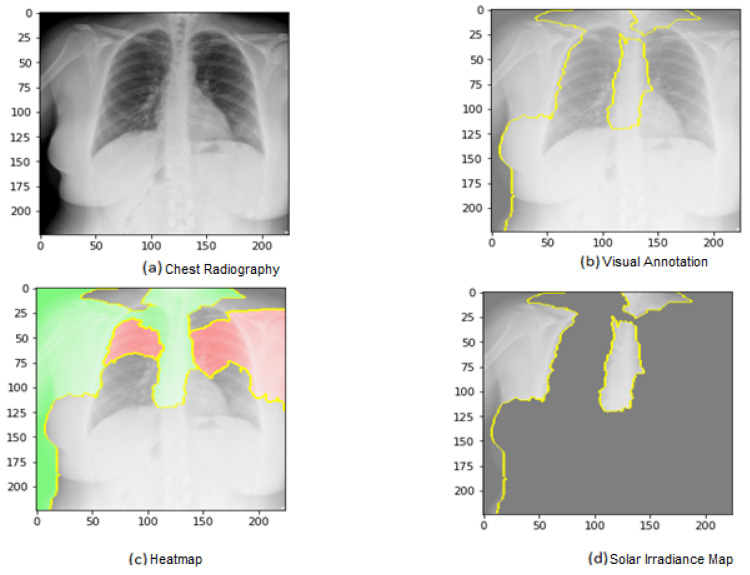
Explainability of X-ray images in the second dataset.
